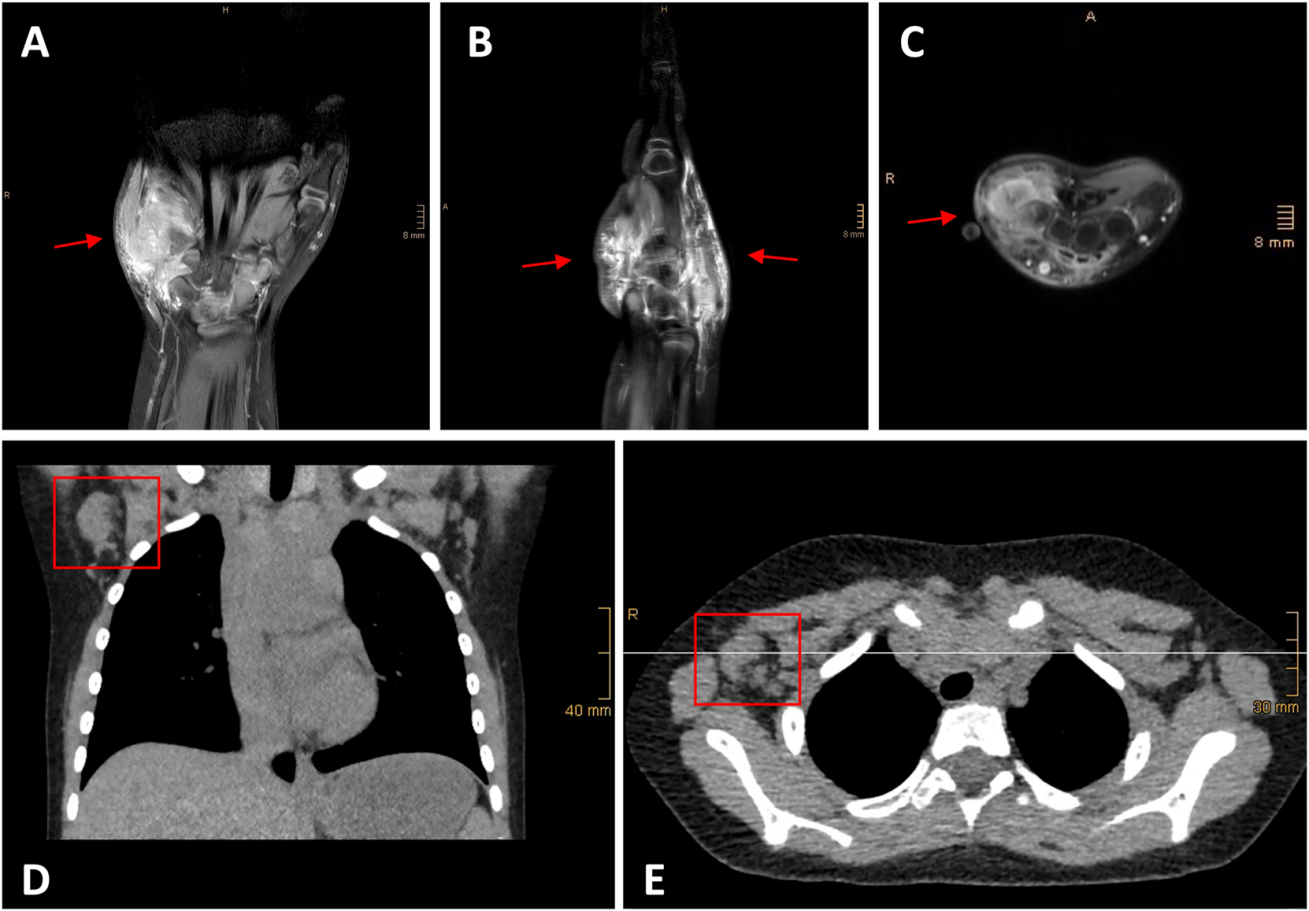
Initial contrast-enhanced MRI pictures of the right hand coronal (A), sagittal (B) and axial (C) view. Computed tomography (CT) scan of the thorax revealed an enlarged lymph node in the right axilla (D coronal and E axial view).
